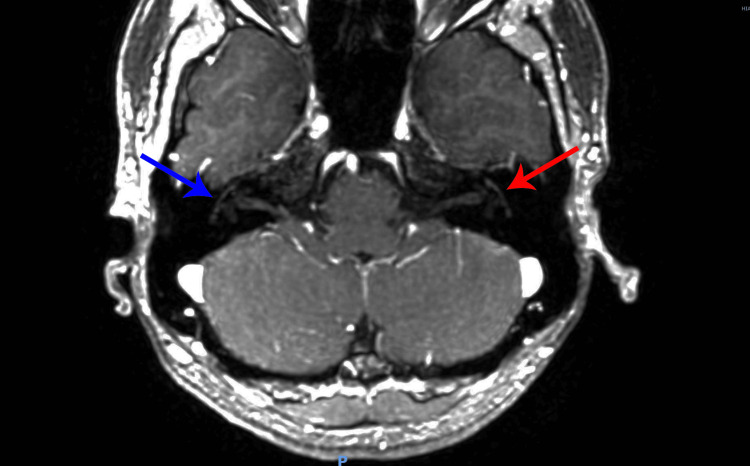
Contrast-enhanced axial MRI performed in February 2023 demonstrates normal right facial nerve (blue arrow) and normal left facial nerve (red arrow).
